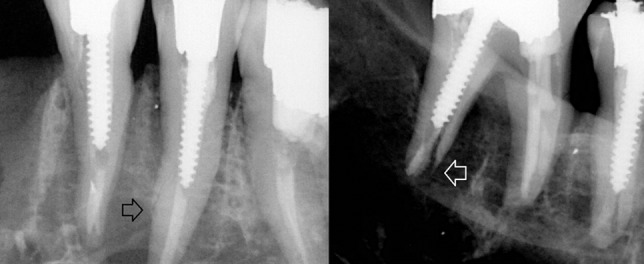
The fracture line in a root-filled and post cemented tooth is detectable (arrow) in these digital periapical radiographs.
